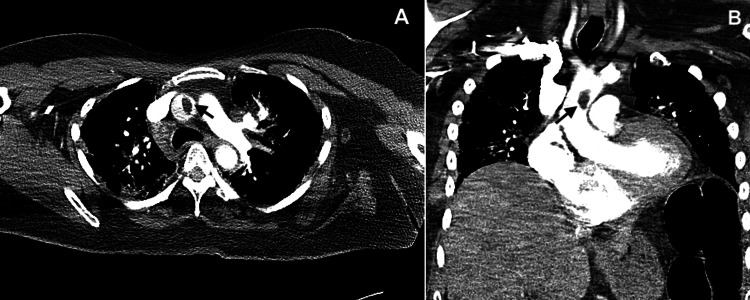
Computed tomography angiography of the chest, axial (A) and coronal (B) slices, showing an intraluminal filling defect (black arrows) in the mid ascending aorta.
